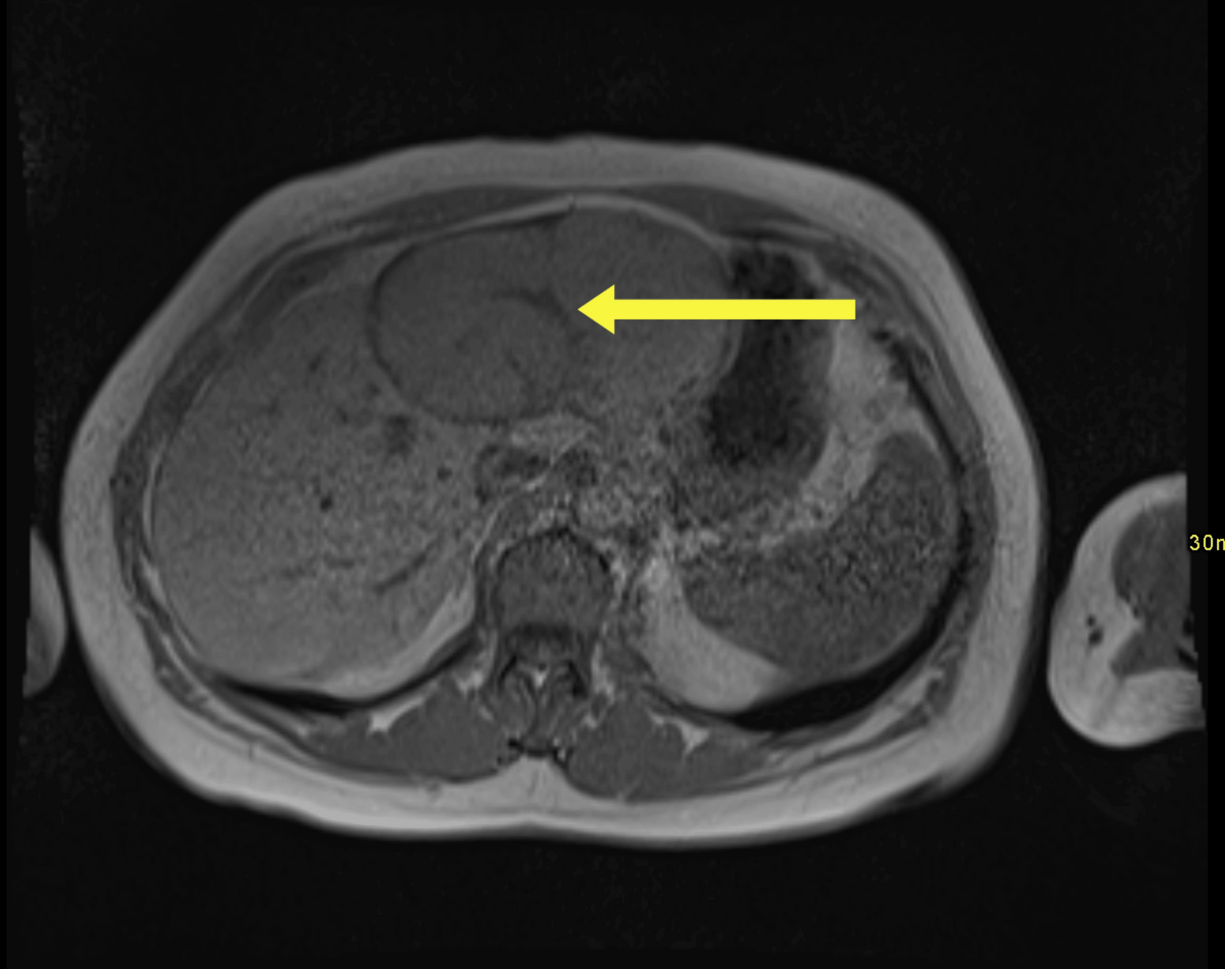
T1-weighted non-contrast image demonstrating a well circumscribed, isointense mass with a central hypointense stellate scar which is characteristic of focal nodular hyperplasia.
